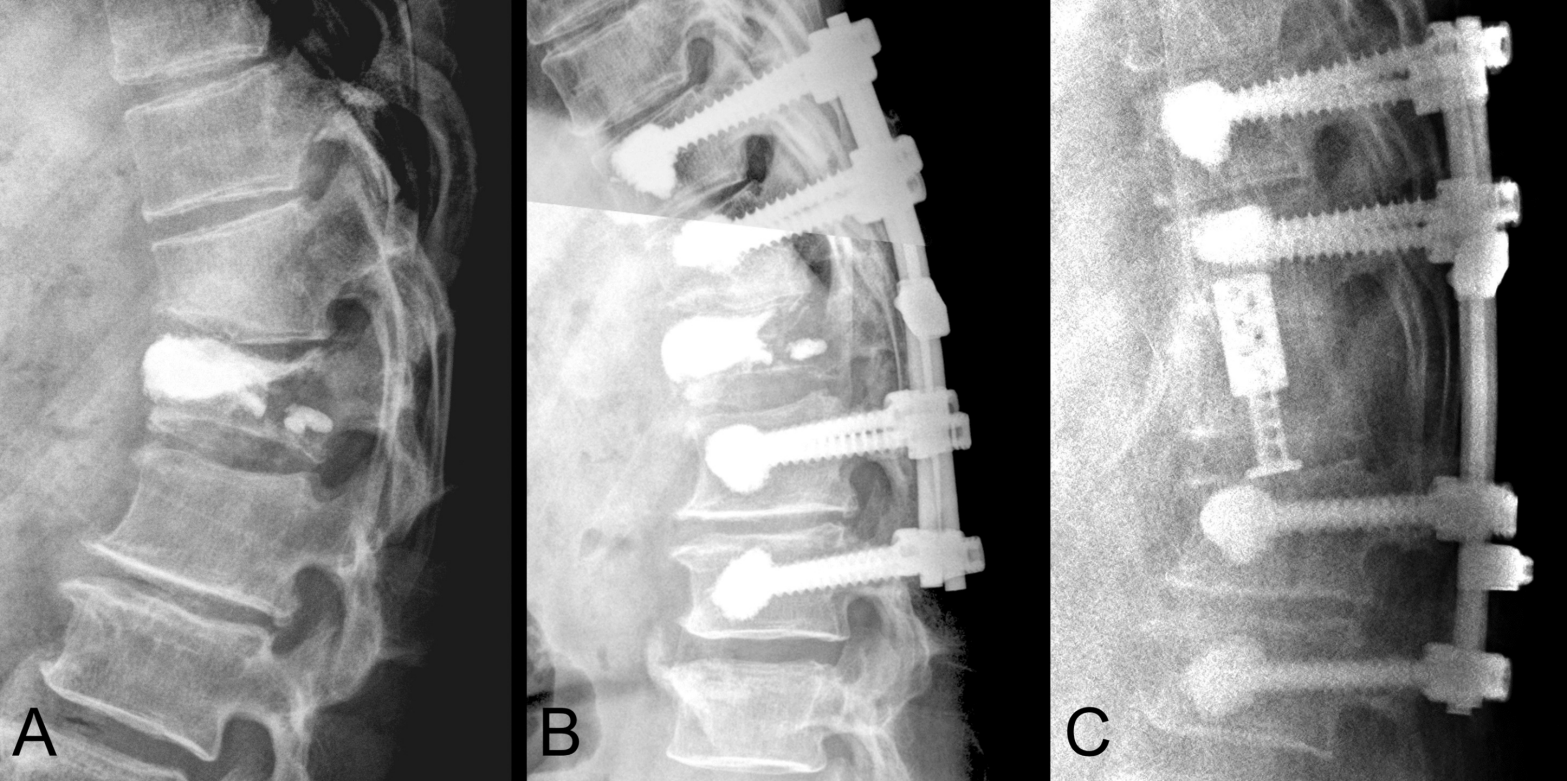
X-ray series of a T12 compression fracture (A) Sagittal view of the lumbar spine radiograph after T12 vertebroplasty. (B) Sagittal view of the lumbar spine radiograph after T12 laminectomy and fixation from T10 to L2. (C) Sagittal view of the lumbar spine radiograph after T12 partial corpectomy, expandable prosthesis reconstruction, and fixation.
